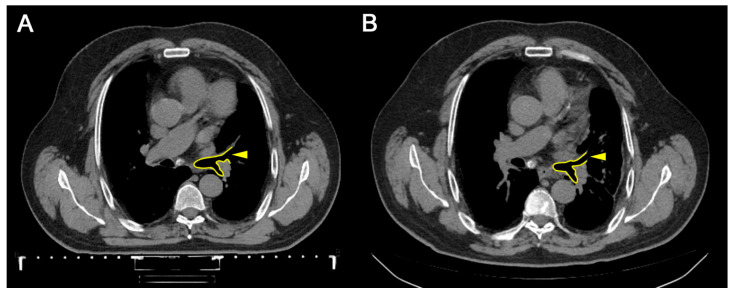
Computed tomography of the chest, axial section, the lumen of the left main bronchus. (A): Before the treatment by immunotherapy with autologous activated lymphocytes in combination with tomotherapy. (B): Three months after the treatment. Lumen is enclosed by yellow contour with a pointer; in dynamics, stabilization of the process is noted (size of the lumen is not changed).
